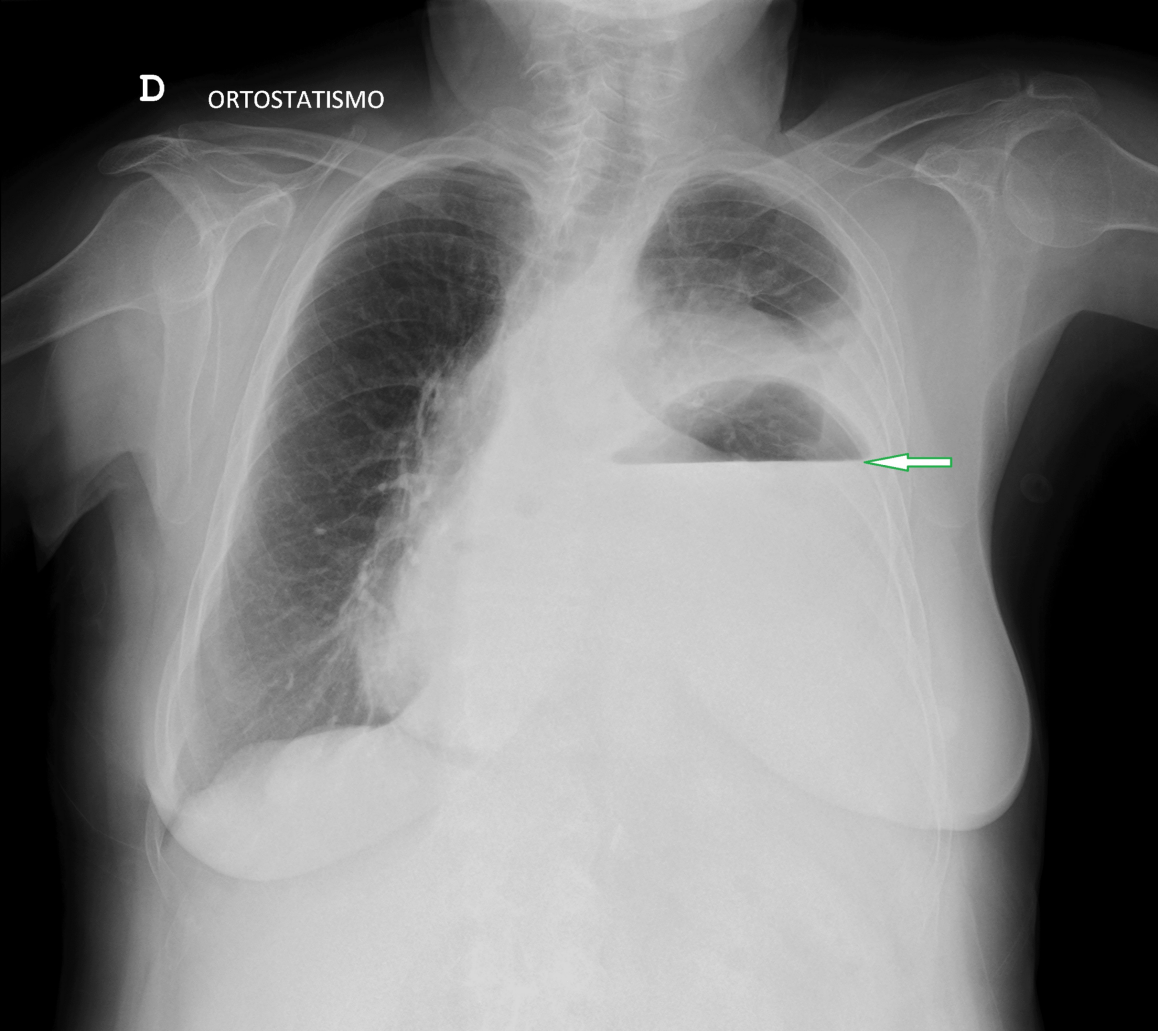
Chest X-ray revealing an intrathoracic gastric air-fluid level (green arrow)
